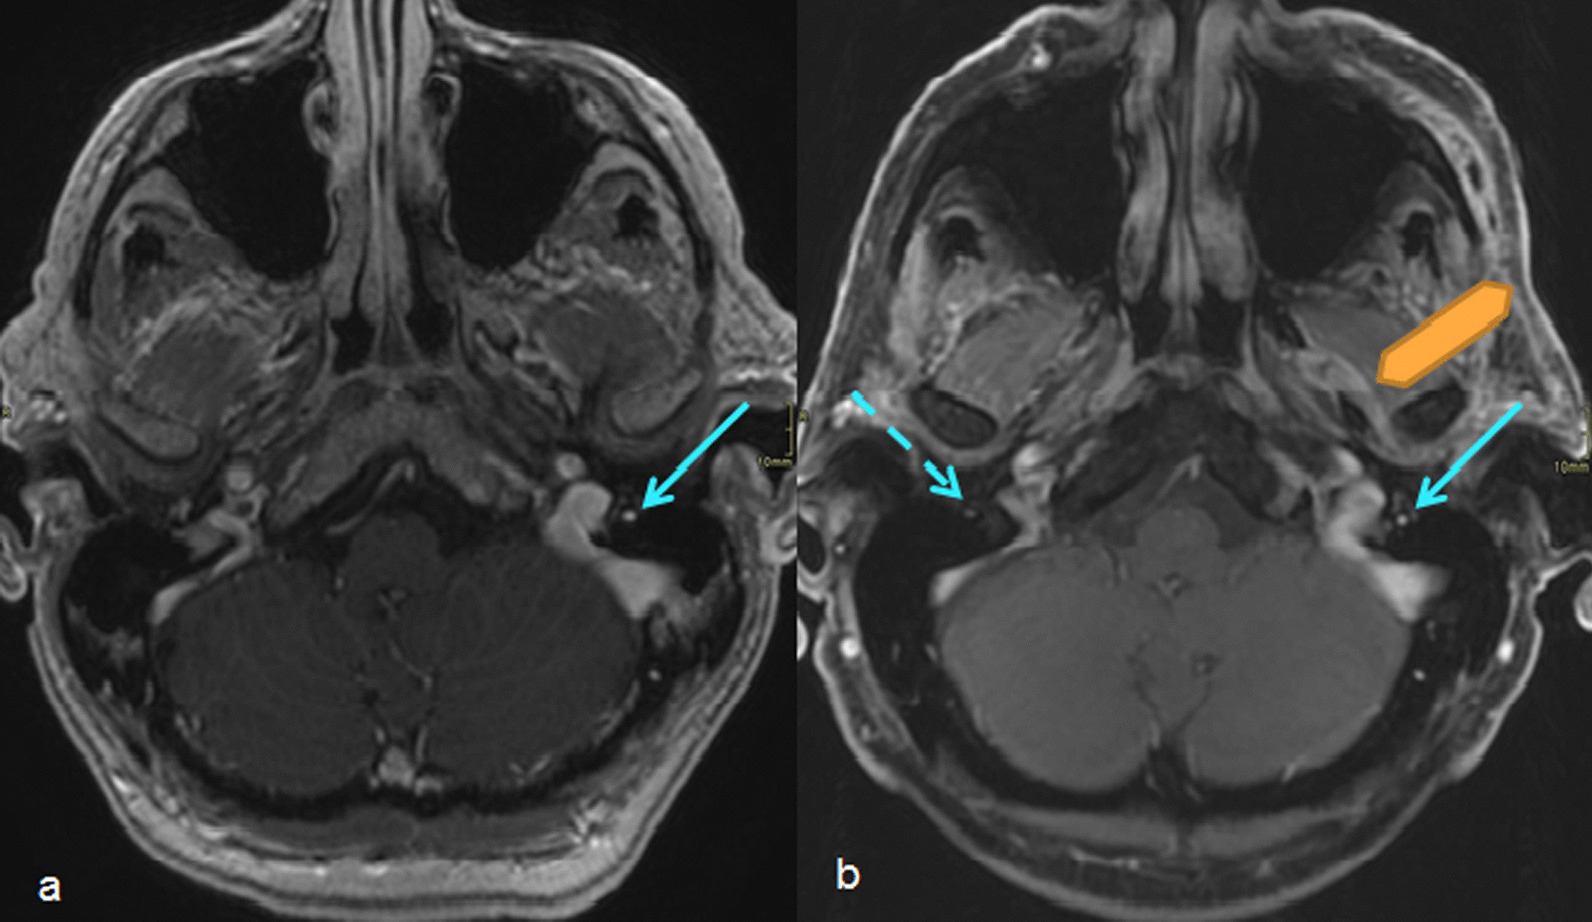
Comparison between initial and follow-up axial T1 Post-gadolinium MR imaging in patient 1 a MRI shows asymmetric enhancement of the mastoid segment of the left CN VII (arrow) b Follow-up MRI 12 months later shows asymmetric enhancement in the left masticator space extending medially along the expected course of the auriculotemporal nerve (double arrow), a branch of the left trigeminal nerve
